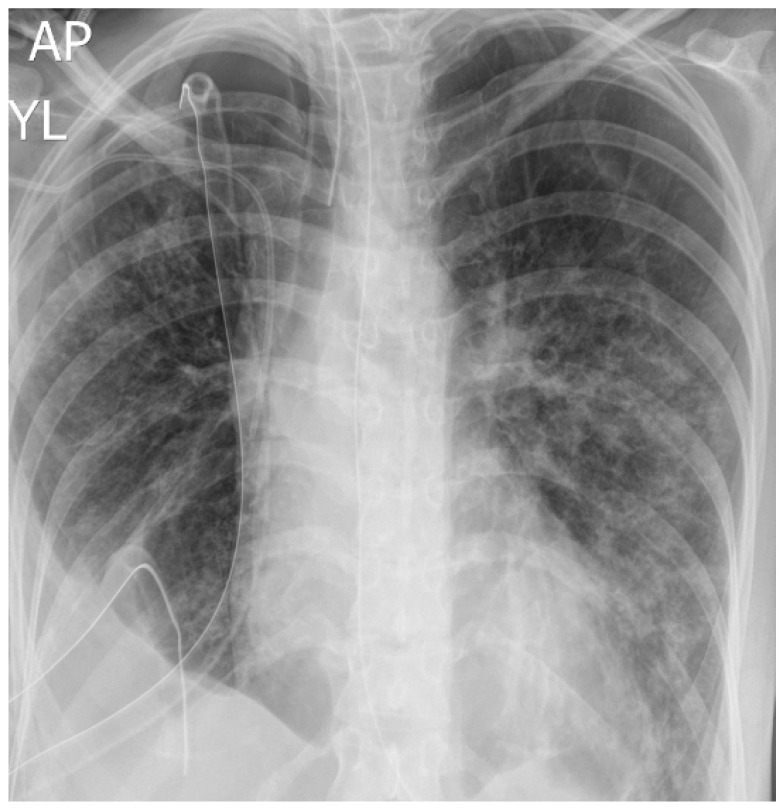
Chest X-ray of patient 1 at admission to the intensive care unit.
